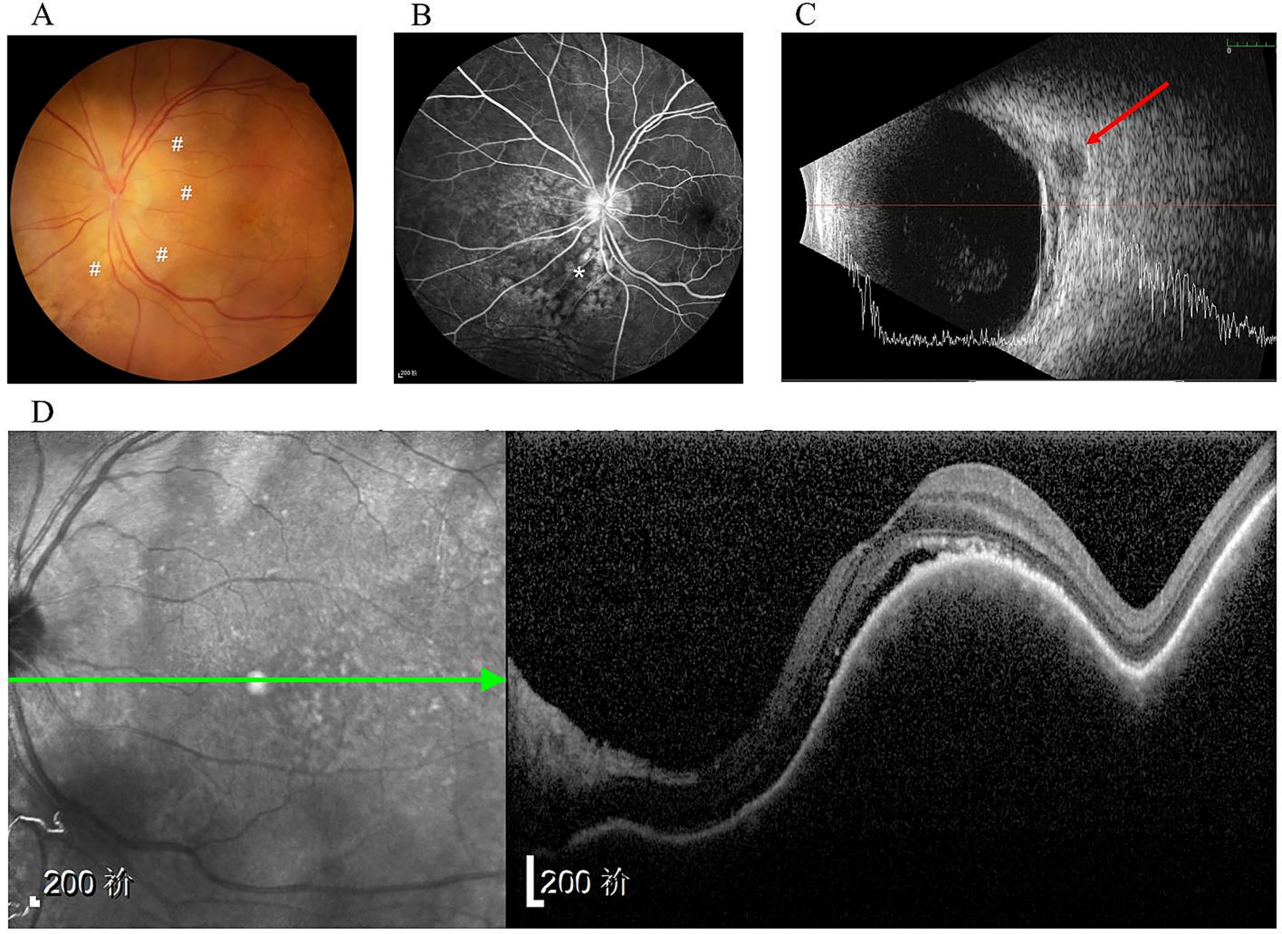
Initial presentation. (A) Fundus examination showed an elevated yellow-white sub-retinal infiltrates (#); (B) FFA taken at 3 min showed mottled hyper-fluorescence area (*); (C) Ultra-sonography showed diffuse choroidal thickening and retrobulbar hypoechoic nodule (arrow) in the left eye. (D) OCT of the left eye showed domed choroid thickening on the macula.
